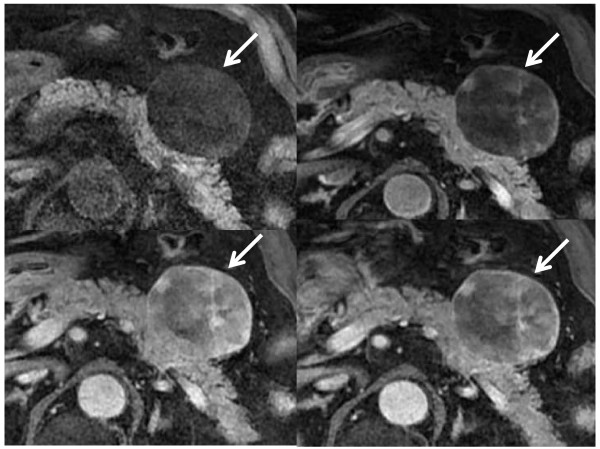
Dynamic MR images show gradually increasing enhancement of the tumor after IV adminsitration of gadolinium-DTPA. Pre-contrast (top left); post-contrast, 30 sec (top right); 60 sec (bottom left); and 90 sec (bottom right).
